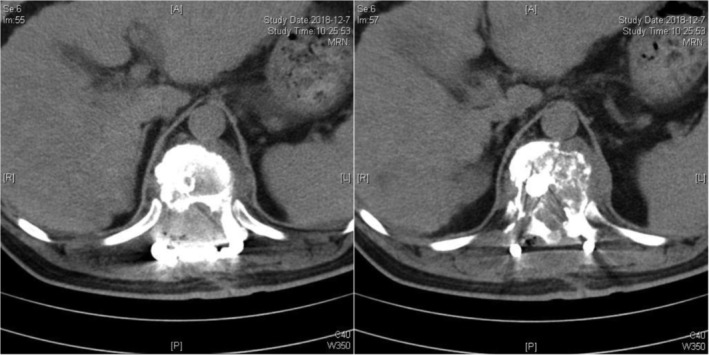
CT Scan after SBRT precision radiotherapy (2 months).
